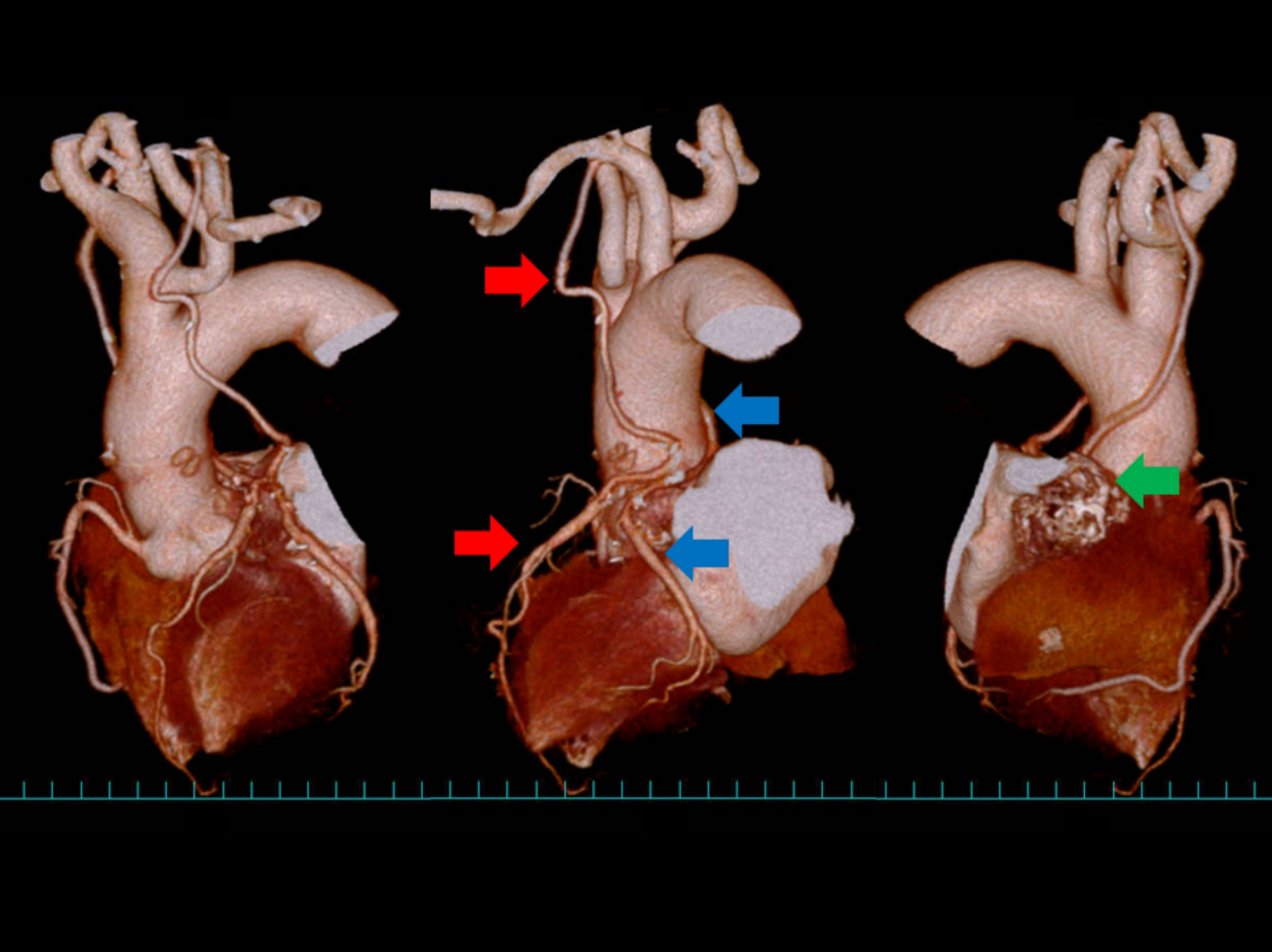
One year after surgery. MDCT rotational view (from left to right). Both bypass vessels were intact. Red arrow: LITA-LAD bypass. Blue arrow: RITA-LCX bypass. Green arrow: shrunk aneurysm. LAD, left anterior descending artery; LCX, left circumflex coronary artery; LITA, left internal thoracic artery; MDCT, multidimensional computed tomography; RITA, right internal thoracic artery.
